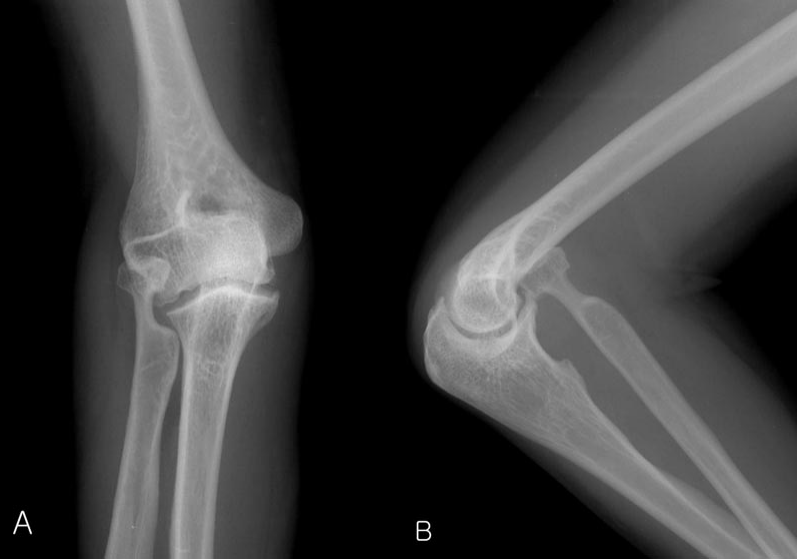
Plain radiographs showing anterior dislocation of the radial head and elongated radial neck: (A) anteroposterior view; (B) lateral view.
